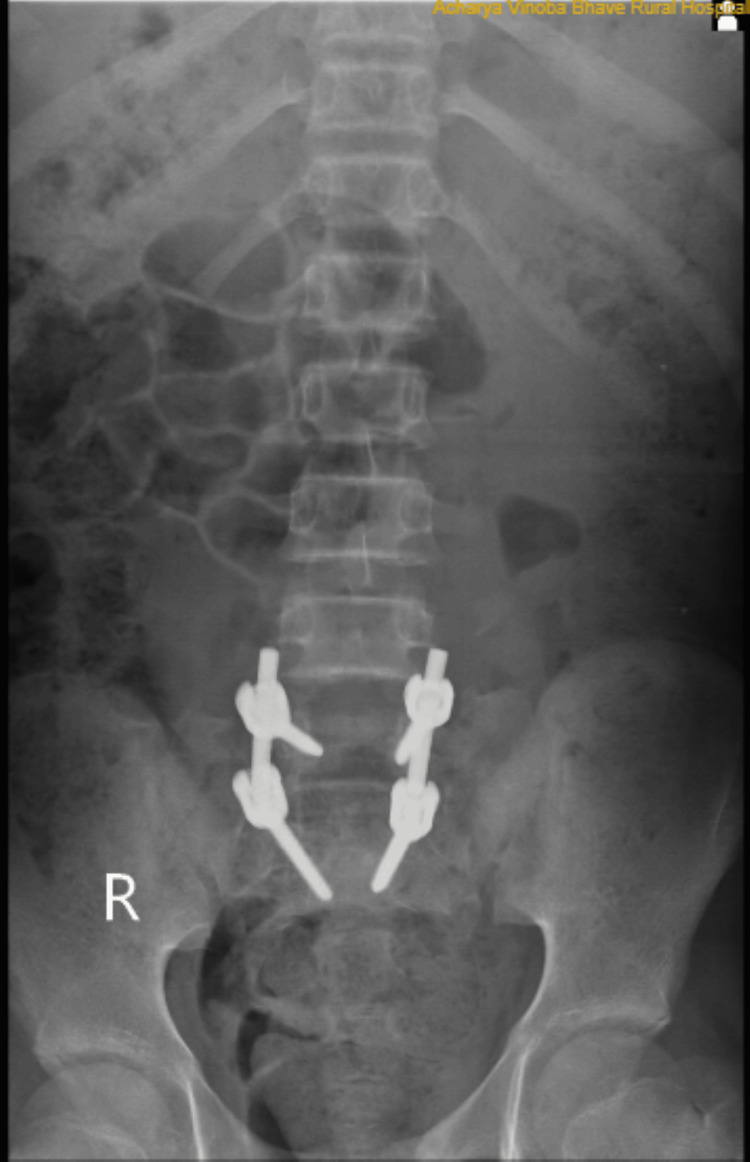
Post-operative anterior-posterior X-ray of the lumbar spine, showing spine fusion of the L4-S1 vertebrae
